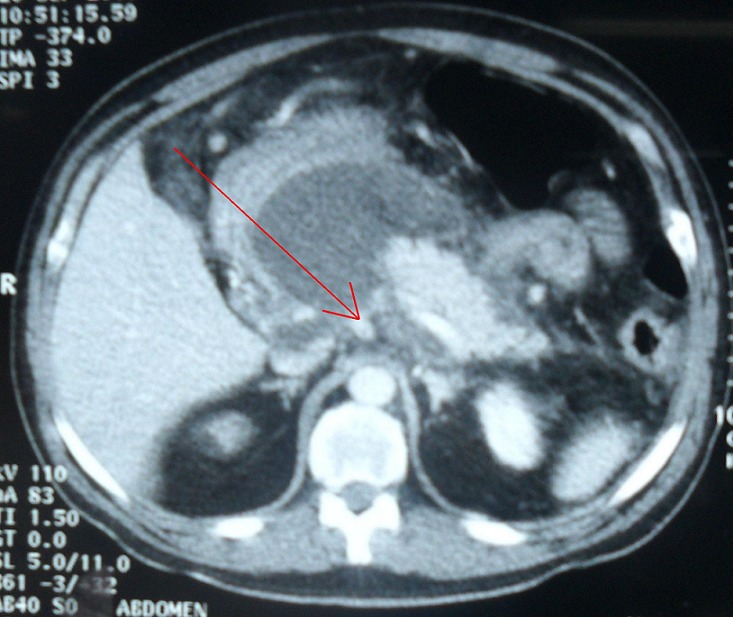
CT scan: A 135x58 mm well circumscribed unilocular cystic lesion, this lesion adhered to the posterior wall of the stomach and compressed the superior mesenteric artery (arrow)
